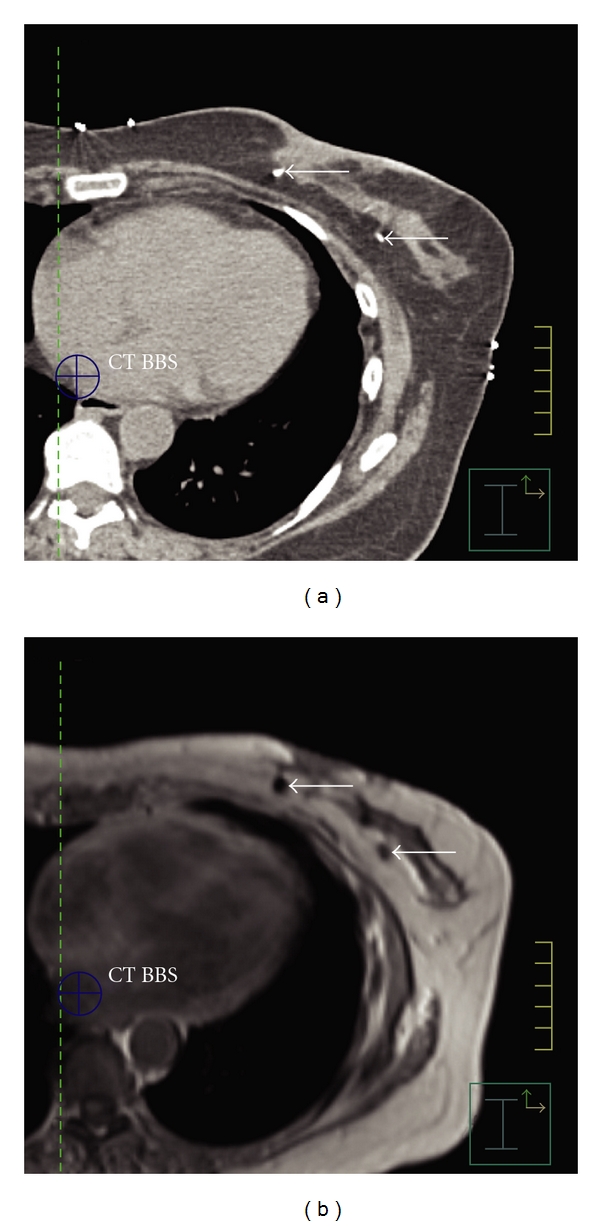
(a) Biopsy clips visible on CT. (b) Biopsy clips seen as signal void on T1 MRI.
